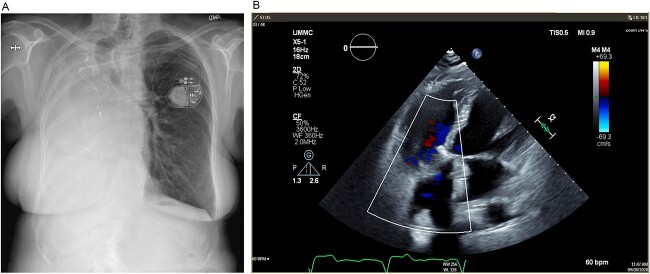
(A) Postoperative posteroanterior view chest X-ray. (B) Postoperative transesophageal echocardiogram with doppler showing a well-seated bioprosthetic valve in the tricuspid position with a mean gradient of 4 mmHg and no measurable insufficiency.
